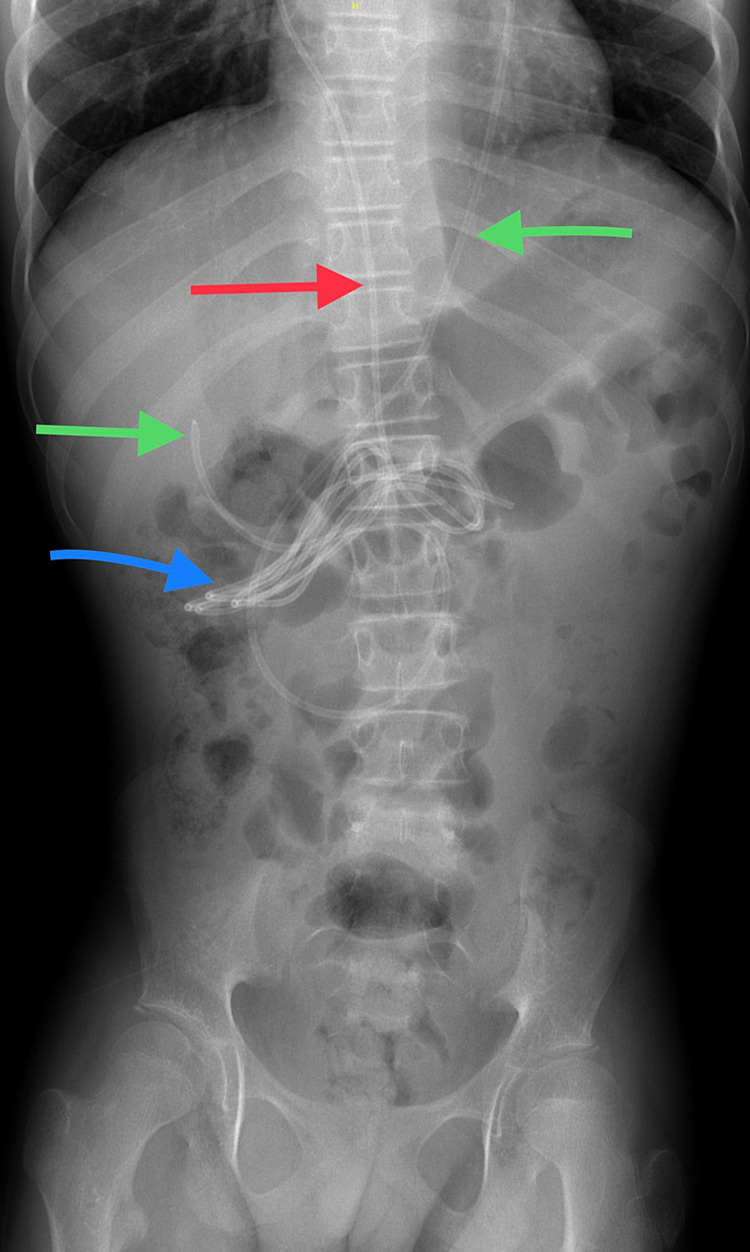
X-ray abdomen AP view - the red arrow refers to the new distal catheter, the green arrows refer to the old distal catheter, and the blue arrow refers to an abnormal multiple transverse looping of the new catheter at the transverse colon.
